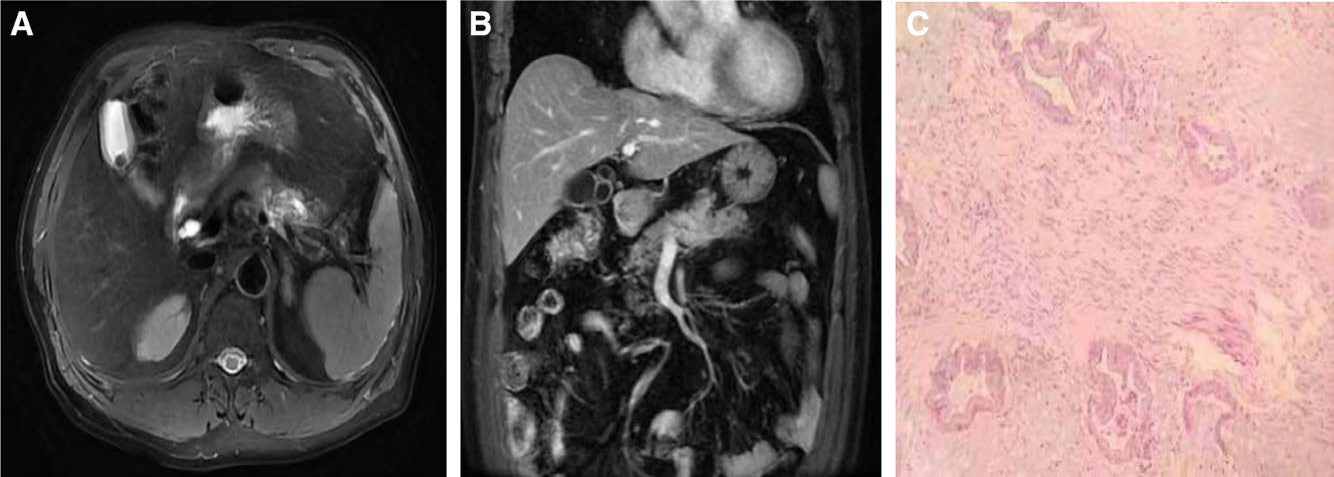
Primary pancreatic tumor. Contrast-enhanced CT scans (A. The abdomen in transverse view, B. coronal plane of the abdomen) showed that a pancreatic mass was mainly located in the body and tail of the pancreas, adjacent to the left gastric artery and spleen artery. (C) Pathology confirmed the pancreatic ductal adenocarcinoma (PDAC). CT = computed tomography.
